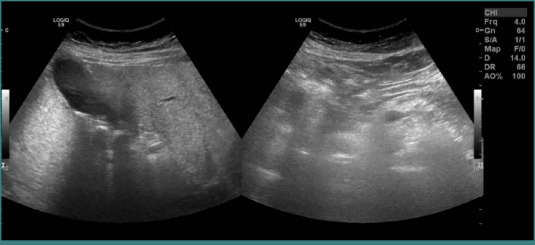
An abdominal ultrasound (US abdomen). The gallbladder contains multiple stones and sludge without mass as a pre-operative assessment.
